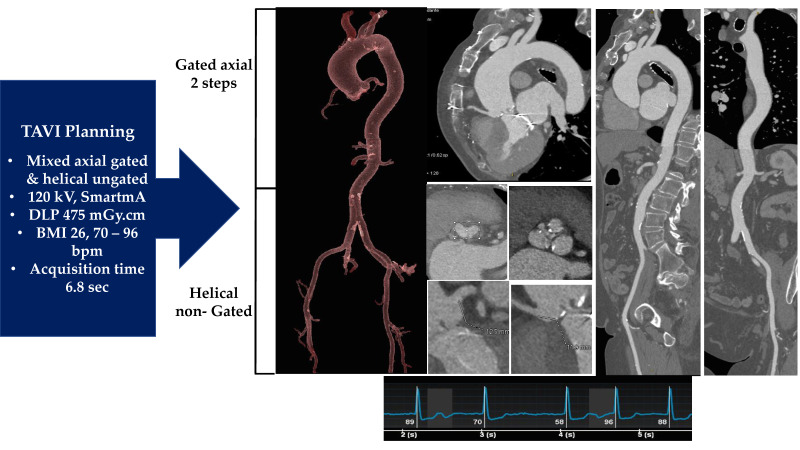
The 3D CT scan protocol for patients receiving TAVI. It is designed to ensure all steps of the transcatheter procedure. The figure reports an example in which the exam was conducted in 6.8 s with only 640 mGy.cm. Precise planning to support the intervention was conferred. Abbreviations; DLP, Dose Length Product; mGy, microgray.
